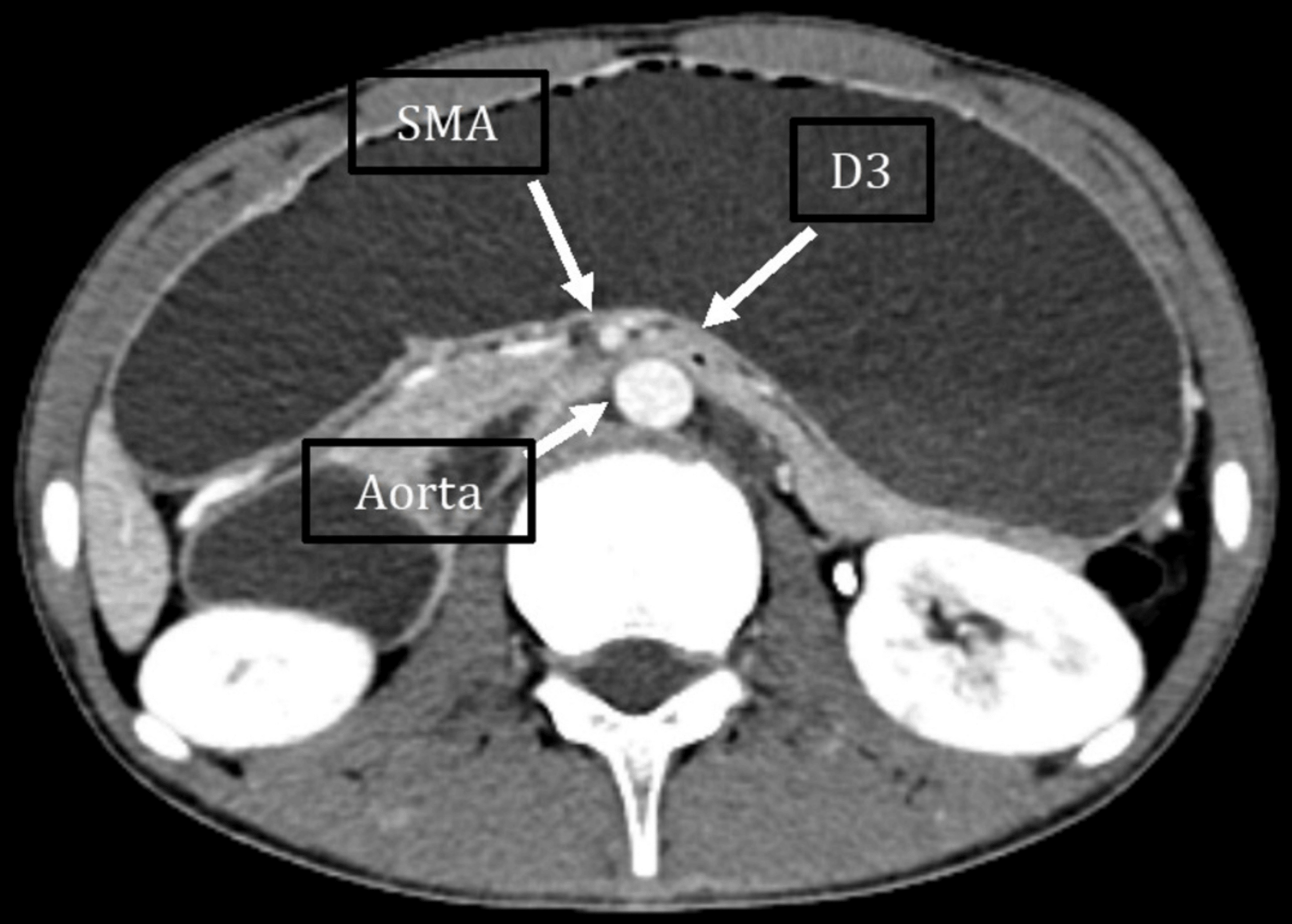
Contrast-enhanced computed tomography (CT); axial reconstruction of the abdomen shows extrinsic compression of the third portion of the duodenum between the superior mesenteric artery (SMA) and the aorta, with the aortomesenteric distance reduced to 3.9 mm.
